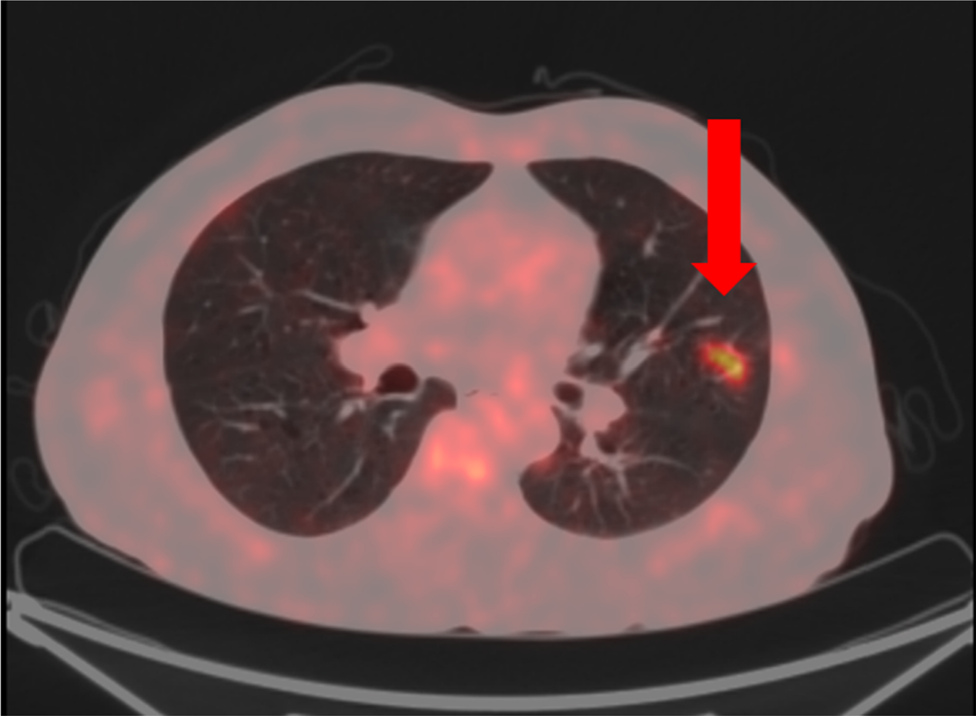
18F-FDG PET/CT image revealed a nodule (1.8 cm × 0.8 cm) in the lingula segment of the left upper lobe (arrowhead).
